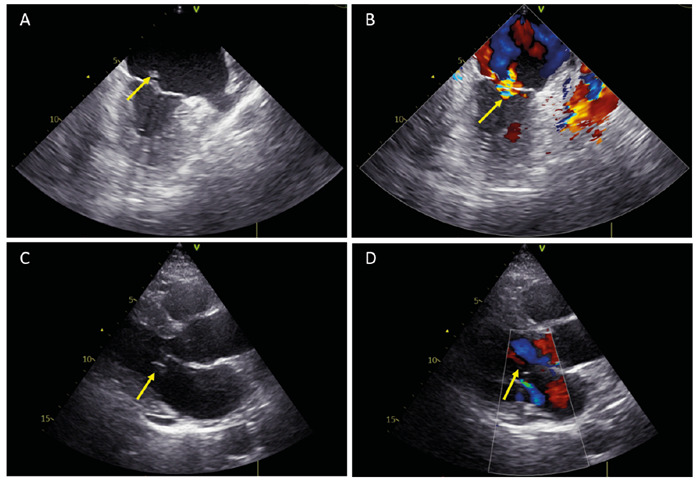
(A) Atransesophageal echocardiography image consistent with vegetation on the anterior mitral valve (1.2 x 0.4 cm). (B) A transesophageal echocardiography image consistent with mild mitral regurgitation. (C) A transthoracic echocardiography image consistent with vegetation on the anterior mitral valve (1.2 x 0.4 cm). (D) A transthoracic echocardiography consistent with mild mitral regurgitation.
